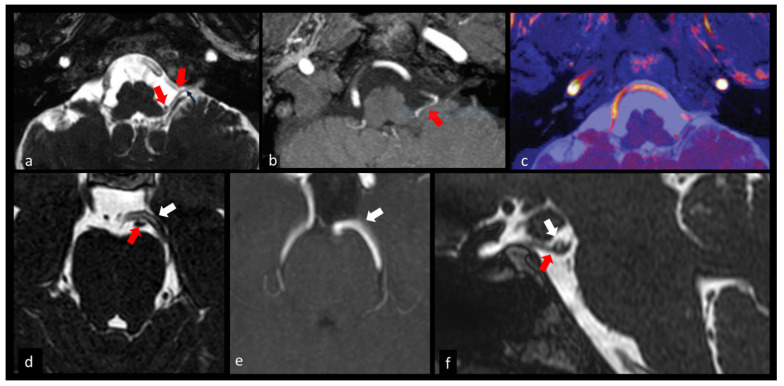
Neurovascular conflicts. (a–c) 71-year-old female with a 3-year history of intense pain localized in the pharynx and the posterior portion of the tongue. Axial 3D CISS (a) and 3D time-of-flight magnetic resonance angiography (3D TOF MRA) (b) show that the left posteroinferior cerebellar artery (red arrows) impacts the IX, X and XI cranial nerves at the root entry zone and along the intracisternal nerve tract. Postprocessed fused 3D CISS-TOF image (c) simultaneously displays the offending vessel (red color) and nerves (blue color). These images were released under CC license (with unrestricted use) (Alafaci et al. [25]). (d–f) Transient left third nerve palsy presumably caused by neurovascular compression. Axial (d) and sagittal (f) 3D CISS images show a close relationship between third nerve (red arrow) and posterior cerebral artery (white arrow) on the left side, with mild inferior displacement of the third nerve. 3D-TOF with maximum intensity projection (MIP) reconstructions (e) depicts the course of left posterior cerebral artery well. The patient spontaneously recovered from symptoms two days after the execution of magnetic resonance imaging (MRI).
